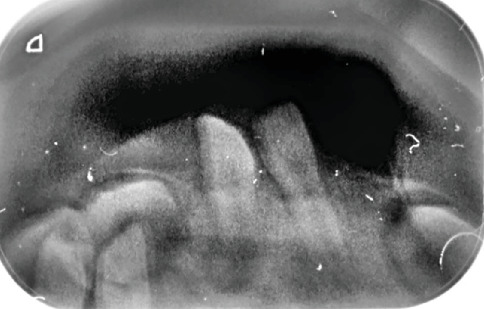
Initial periapical radiograph revealing developing lower incisors. A periapical radiograph taken at the first visit, demonstrating the presence of the lower incisors beneath the gum line despite their clinical absence, thereby confirming ongoing dental development at this early stage.
